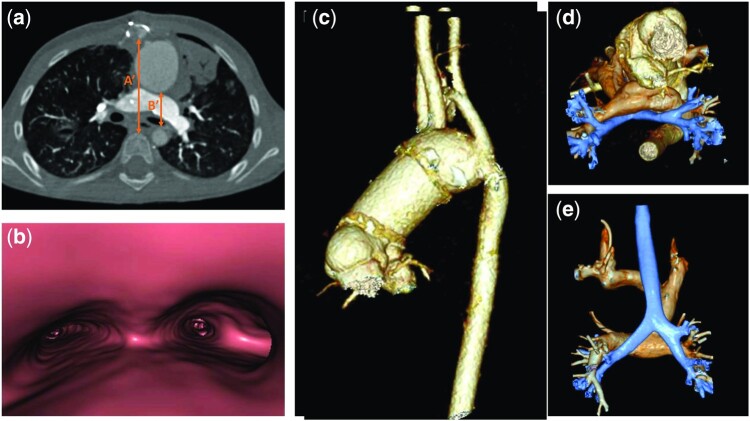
Postoperative computed tomography. The postoperative computed tomography scan (a) shows the appearance of the ascending aorta after surgical elongation with interposition of the Hemashield graft (c-e) and the resulting enlargement of the aortopulmonary space with relief of the main bronchus obstruction, as evident in virtual bronchoscopy view (b). This surgical approach allowed a considerable increase of the interaortic ratio (*B*′/*A*′) compared to the preoperative computed tomography scan.
